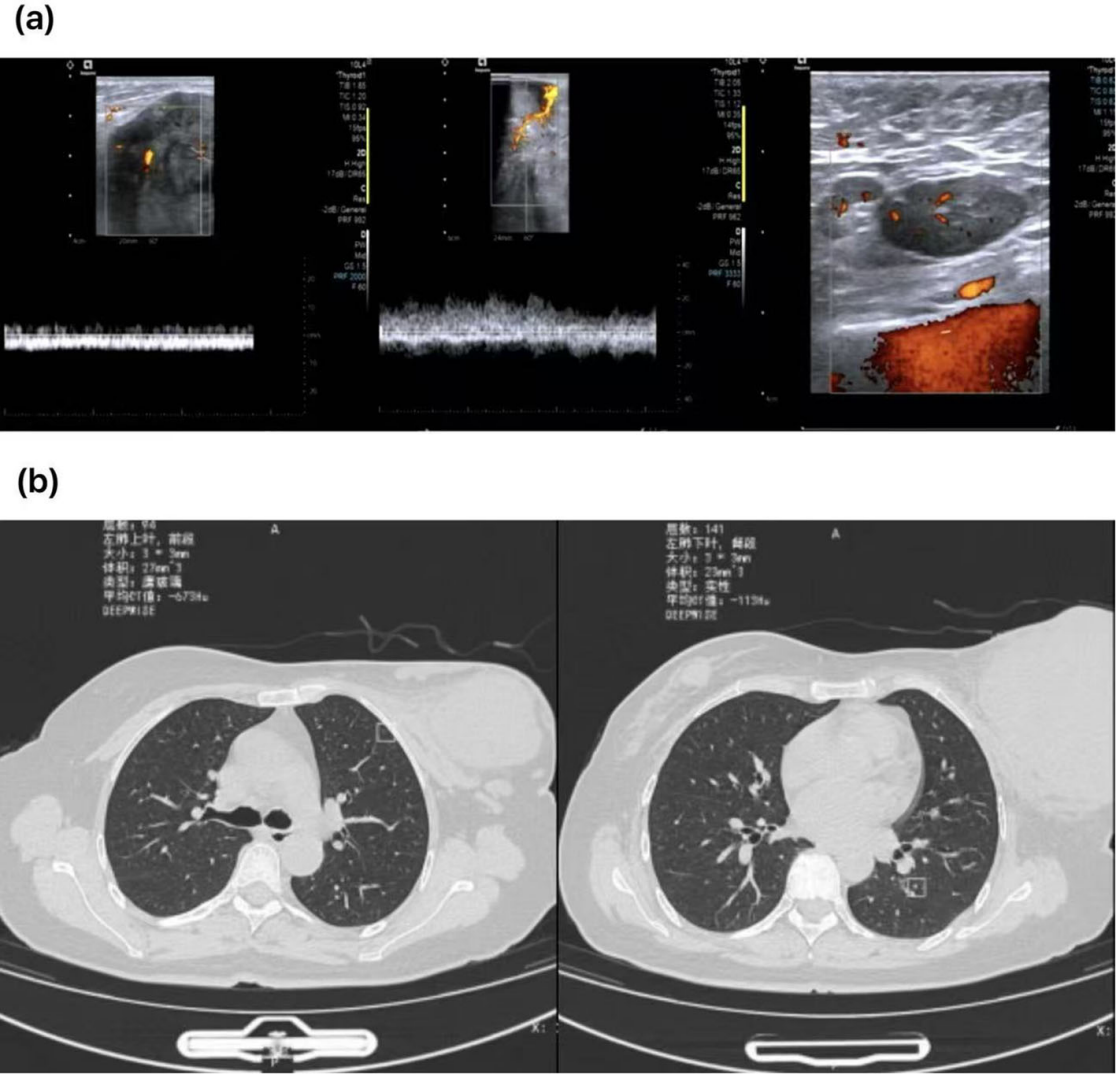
(A) Preoperative ultrasound revealed unclear architecture and abundant blood flow in the left breast.Enlarged left axillary lymph node. (B) A giant soft tissue mass was identified in the left breast, with a small nodule in the right breast. Multiple scattered nodules were noted in both lungs; a low-density shadow was present in the left inner lobe of the liver; and splenomegaly was observed.
